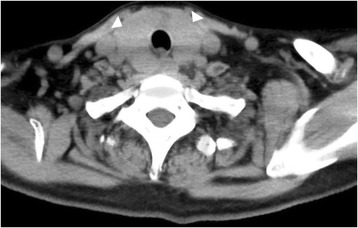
Computed tomographic scan showing thyroid enlargement (*arrowheads*)
